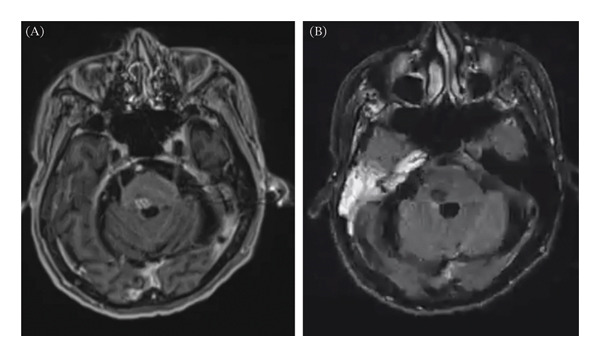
MRI brain at 3 months after initiation of treatment with TB therapy. (A) T1 postcontrast imaging with decreased size of enhancing pontine lesion now measuring < 1 cm. (B) FLAIR imaging demonstrating resolution of vasogenic edema associated with tuberculous lesion.
